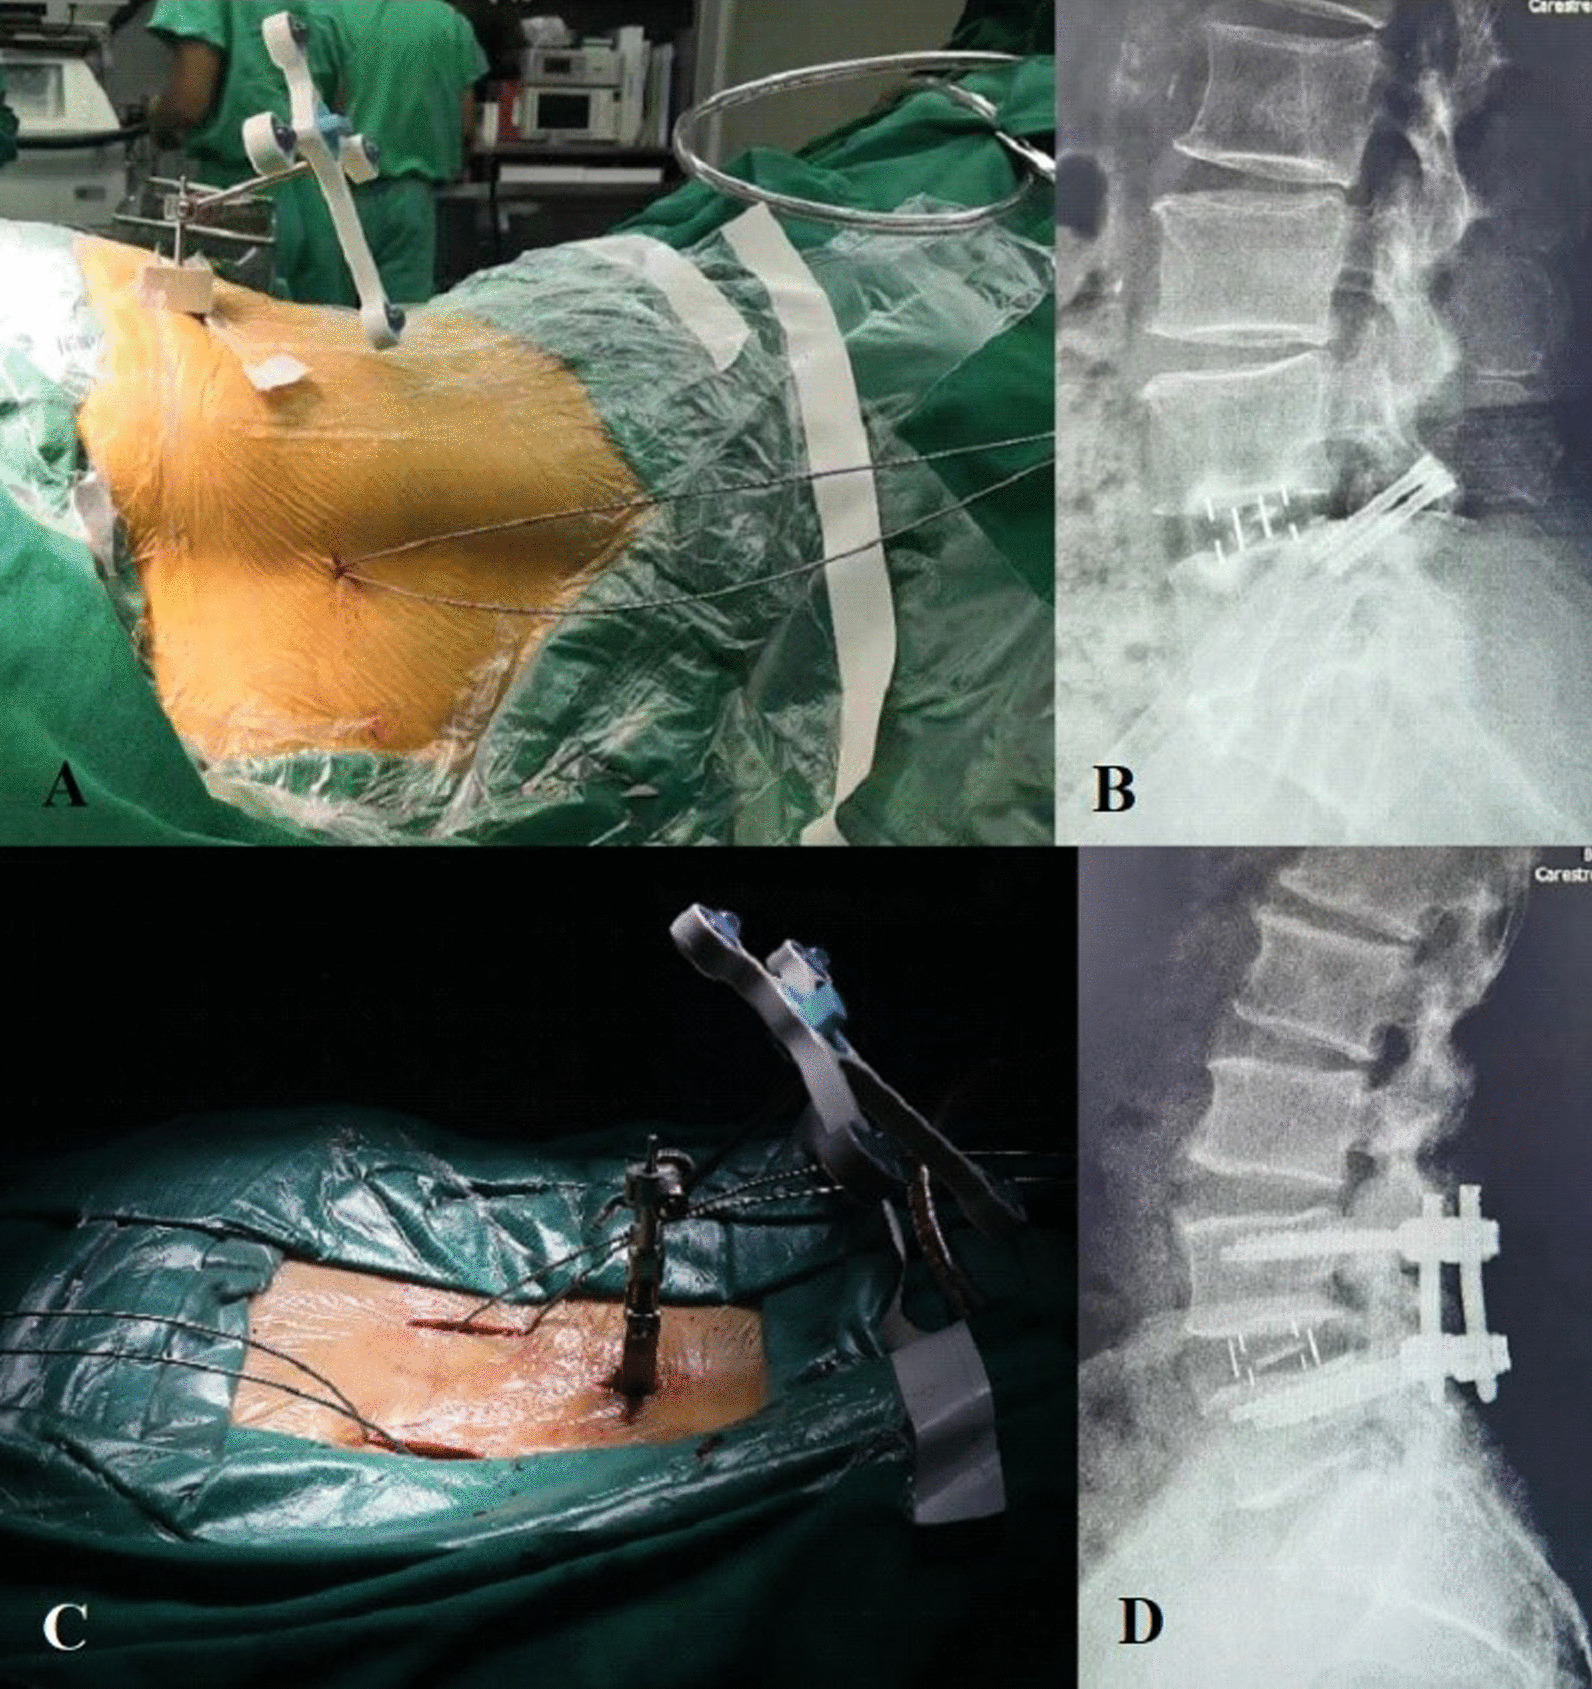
Placement of guiding pins and screws. A and C Guiding pins were placed percutaneously. The incision for TFS was much less invasive than pedicle screws. B and D The lateral X-ray of single-level OLIF surgery supplemented by percutaneous TFS or percutaneous BPS at 12-month follow-up
